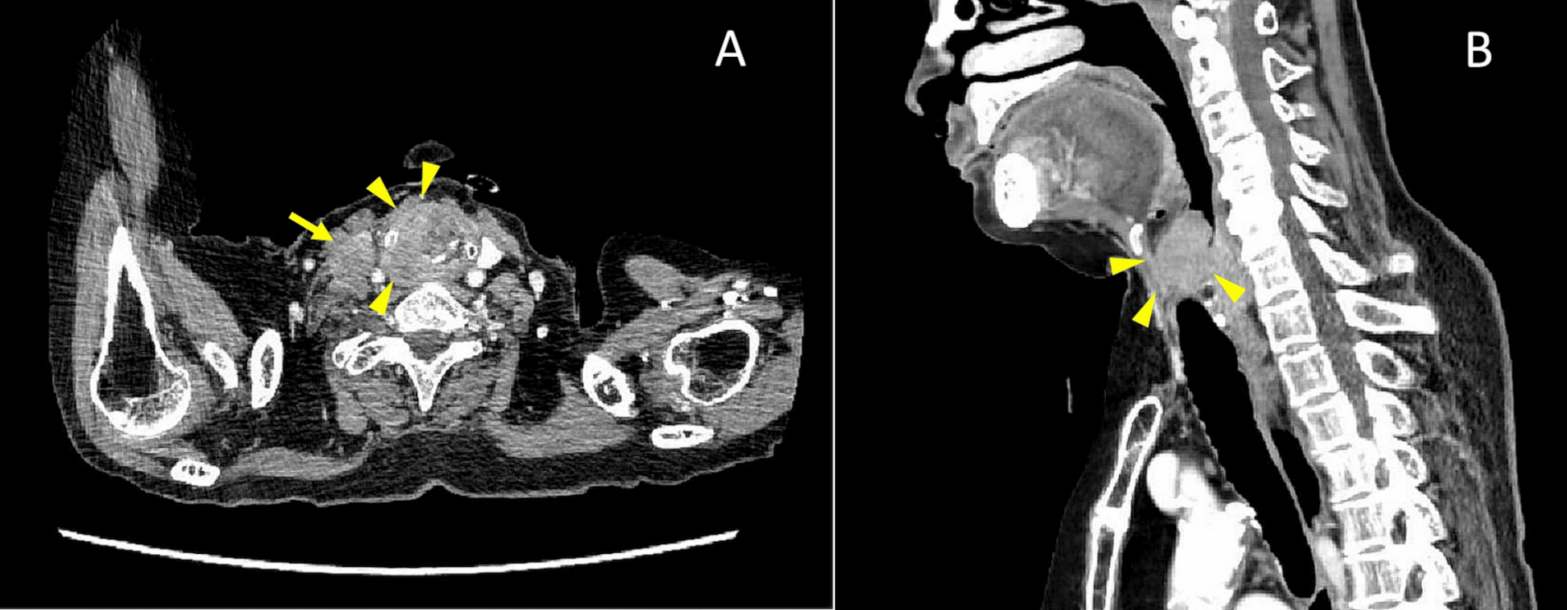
Neck contract-enhanced computed tomography (A: axial; B: sagittal) This reveals a hypopharyngeal mass (arrowheads) with invasion of the thyroid cartilage and enlargement of the right neck lymph node (arrow).
